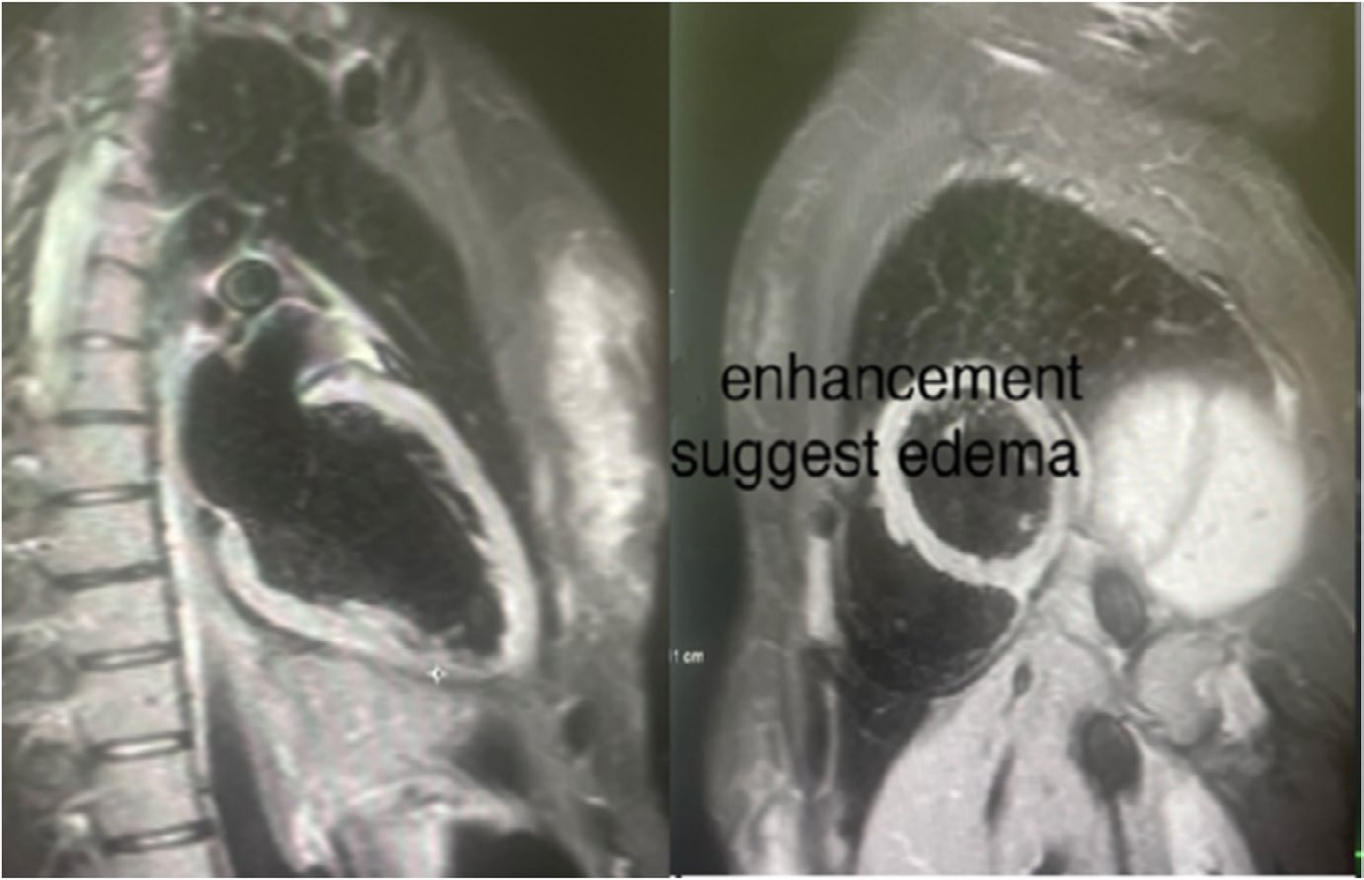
Cardiac MRI showing normal Late Gadolinium Enhancement and mild edema at the inferior wall segment.
